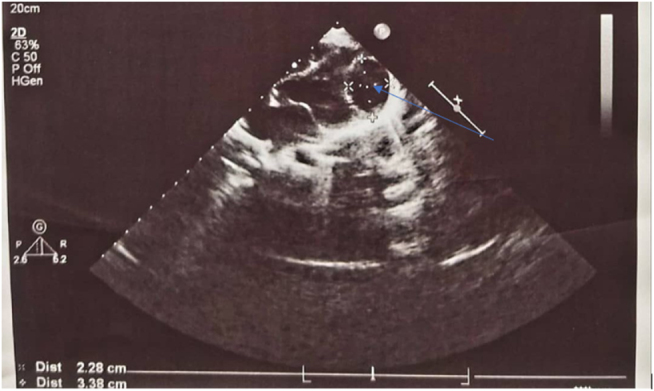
Transthoracic echocardiogram image showing the cardiac cyst (arrow).
